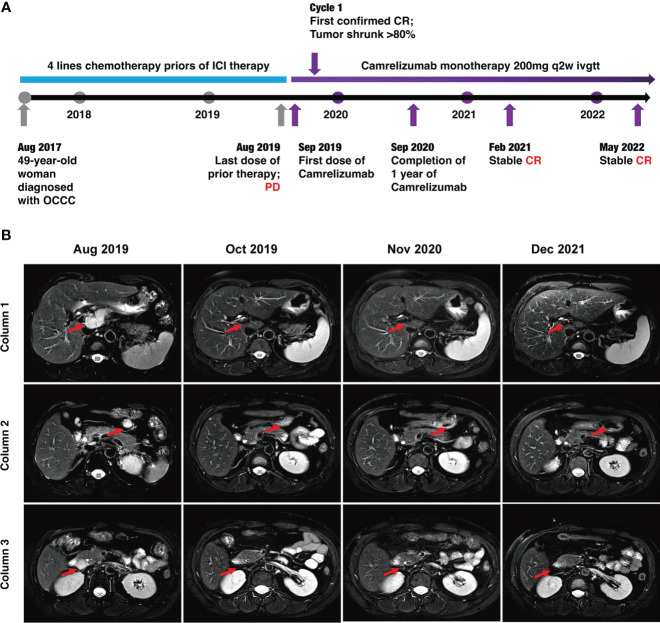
Timeline of the case and MRI findings. (A) Timeline of patient diagnosis, prior therapies, and immunotherapy. (B) MRI scans demonstrating activity (i.e., complete response) to Camrelizumab. The lymph node metastases were located in front of the inferior vena cava (red arrows) in column 1, anterior to the tail of the pancreas (red arrows) in column 3, and around the head of the pancreas (red arrows) in column 3.
